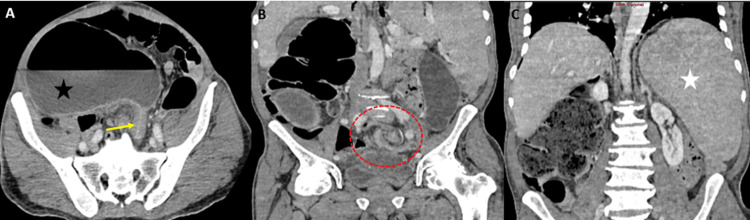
Postoperative contrast-enhanced abdominal CT findings: An important colonic distension (A, black star). A bird beak sign (A, yellow arrow) and a whirl sign with (B, red circle) indicating a mesentericoaxial sigmoid volvulus without any signs of digestive ischemia. A persistently enlarged spleen located in the left hypochondrium following splenopexy (C, white star).
